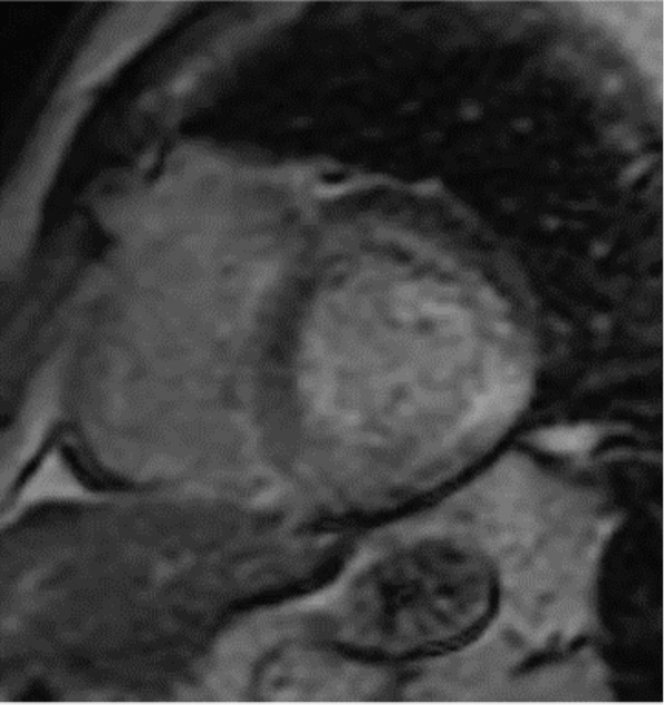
Cardiac Magnetic Resonance Imaging of Patient 2 Showing Late Gadolinium Enhancement of the Basal Midinferolateral, Basal Midlateral, and Basal Inferior Wall
